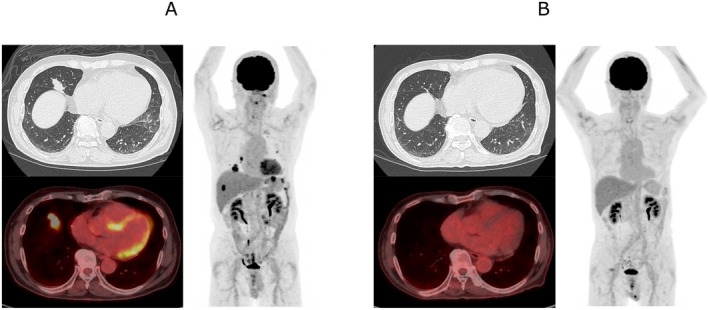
FDG‐PET imaging before and after chemotherapy. (A) Pre‐treatment images showing abnormal FDG uptake in the lung and stomach on fused PET/CT and maximum intensity projection (MIP) images. (B) Post‐treatment images demonstrating complete resolution of abnormal FDG uptake, consistent with a complete metabolic response.
